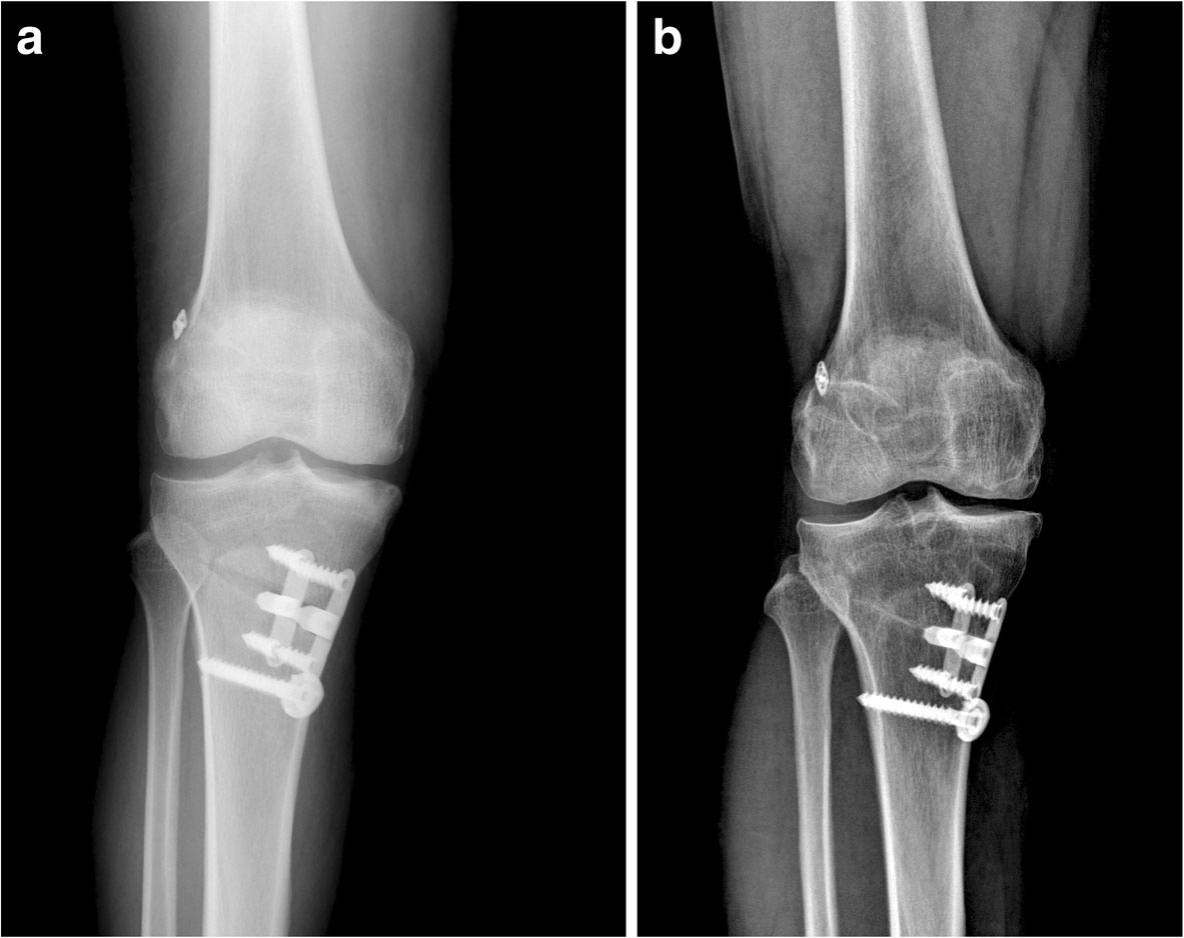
a Immediate operative knee x-ray showing medial compartment osteoarthritis following ACL reconstruction and valgus high tibial osteotomy. b Knee x-ray taken three years following ACL reconstruction and valgus high tibial osteotomy that shows the progression of arthritis of medial compartment
